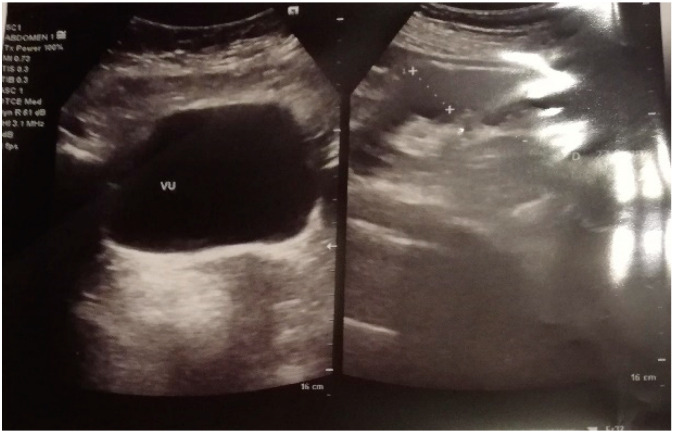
Ultrasound image of bladder, with minimal post-void residue—February 2021 (VU = urinary bladder).
